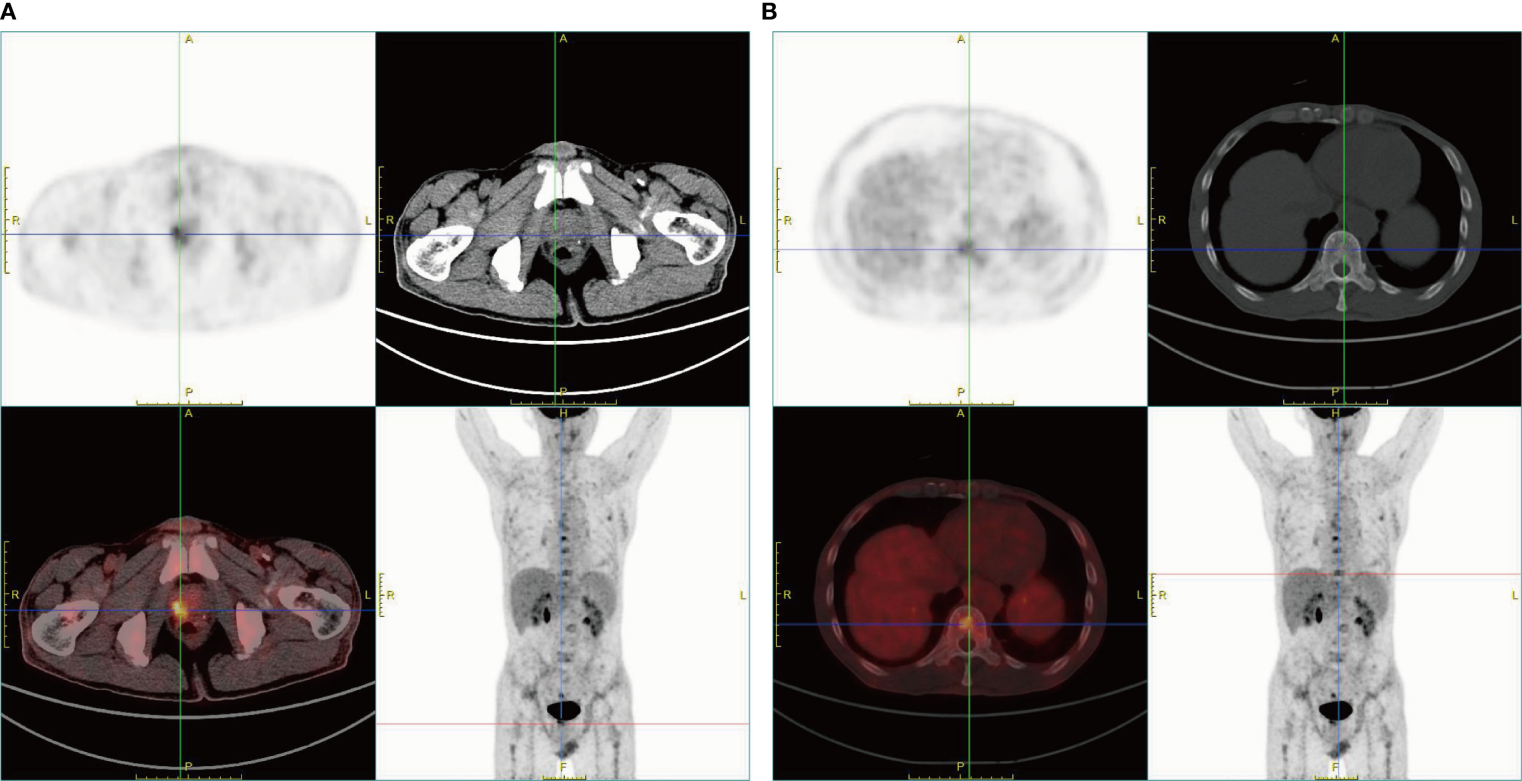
PET/CT images of the patient. (A) The enlarged prostate gland with punctate radiodense shadows within the gland was observed. A focus of increased FDG uptake was identified in the right lobe of the prostate gland, suggesting the potential of prostate cancer. (B) Diffusely increased bone density throughout the skeleton was observed. Foci of elevated FDG uptake in multiple bones were identified, suggesting the potential of bone metastases.
